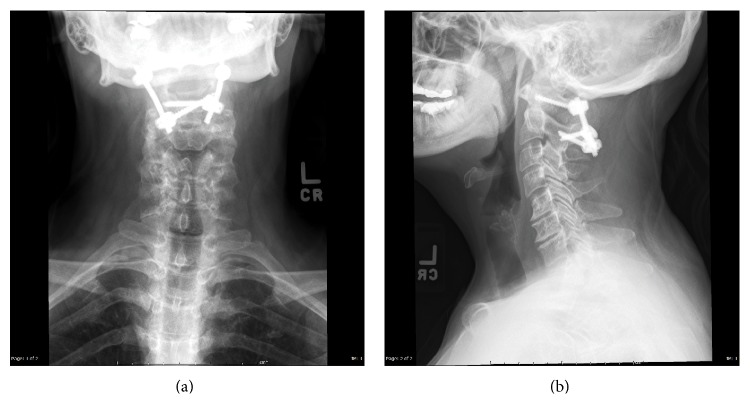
AP (a) and lateral (b) X-rays depicting a C1/2 fusion using C2 translaminar screws.
